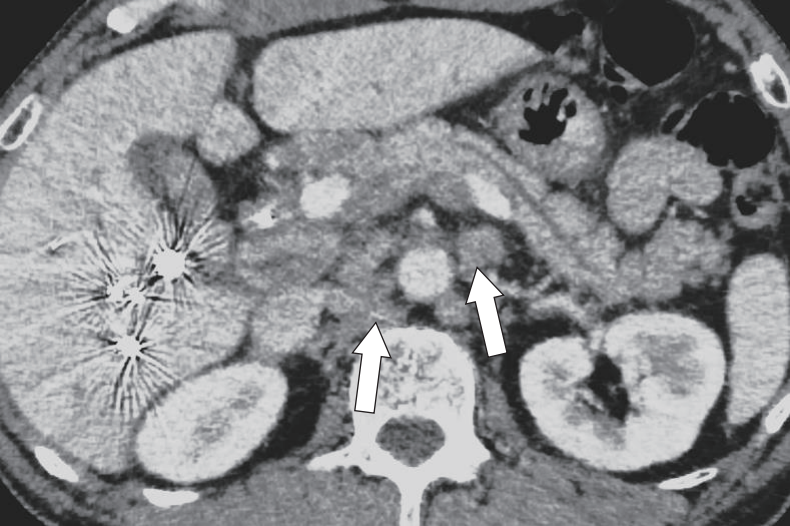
Interval computed tomography scan after right portal vein embolization. The image demonstrates para-aortic lymph node enlargement (white arrows).
